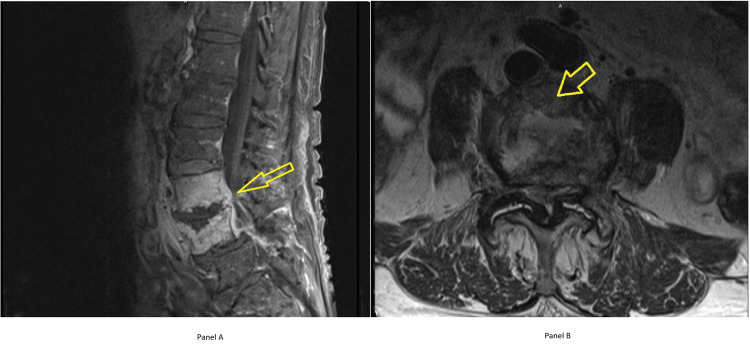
MRI of the lumbar spine Panel A is the sagittal section, in which the arrow highlights marked prevertebral and paravertebral soft tissues edema and enhancement, the degree of which is increased compared to the previous scan; Panel B is the transverse section, in which the arrow highlights destructive process centered at the L3-4 intervertebral disc space with vertebral endplate erosions. These findings have worsened when compared to the previous MRI.
